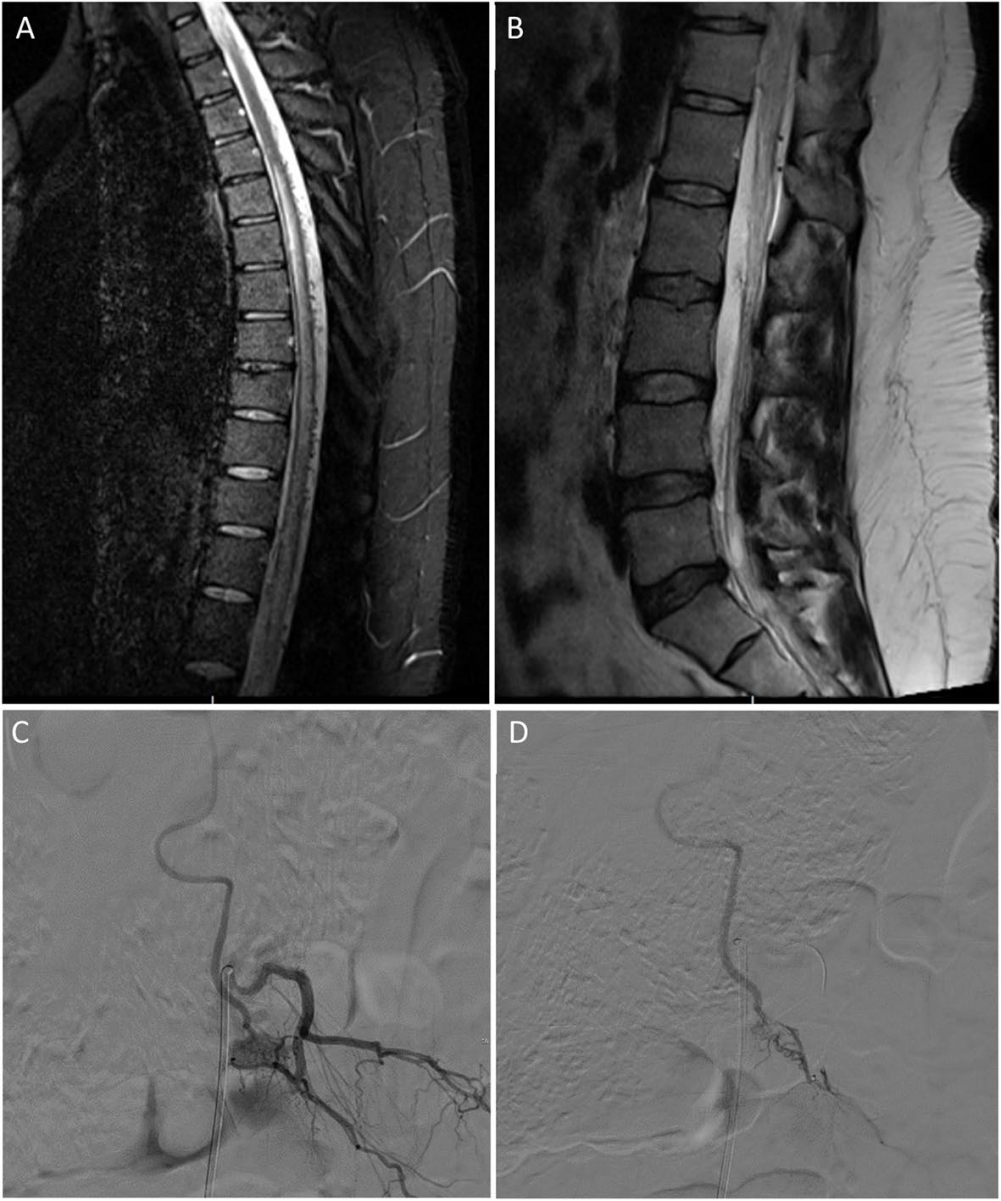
A, B Sagittal thoracic and lumbar spine MRI demonstrating diffuse long segment spinal cord signal abnormality noted predominantly central in location with mild cord expansion seen starting from T4 vertebral body up to the conus medullaris. The cord shows a central hyperintense signal with peripheral hypointensity and multiple vessels surrounding the cord intradural in location along the lower thoracic spine. C, D Angiogram images of a left L2 dural AVF (diagnostic run and selective microcatheter run). Embolization was performed using glue
